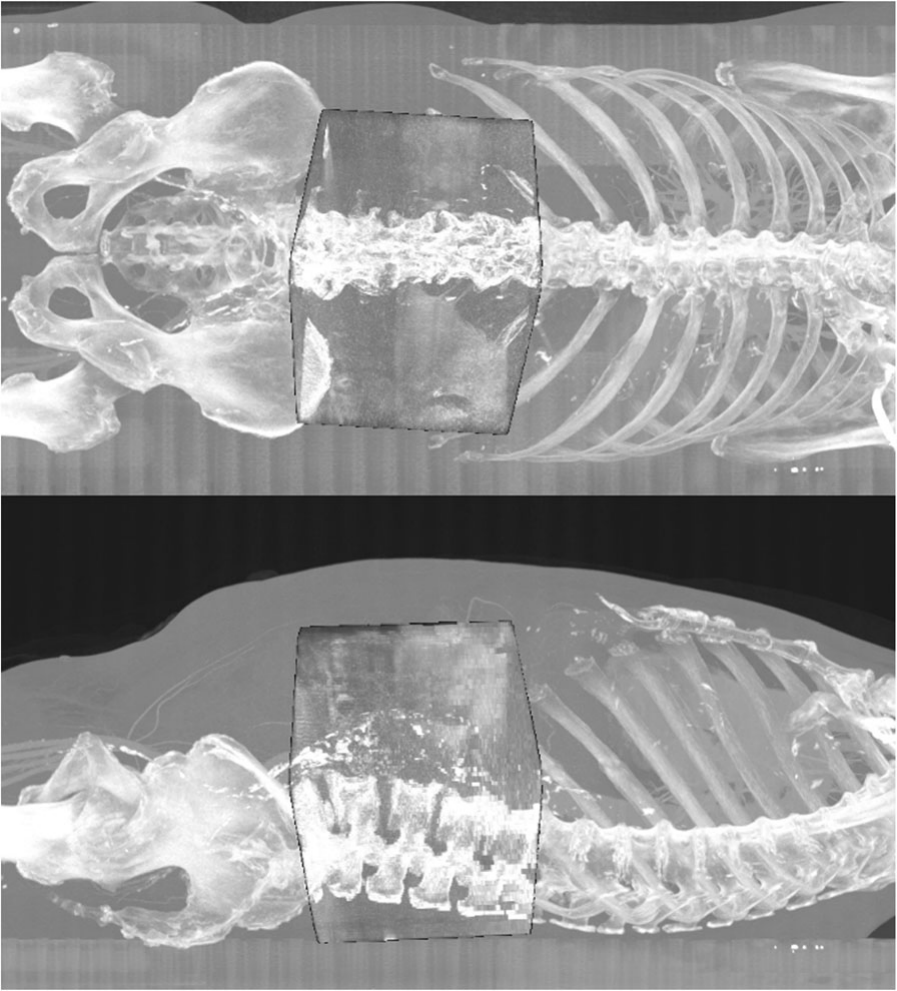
Fused CBCT-CT pair after fully automatic feature-based image registration
